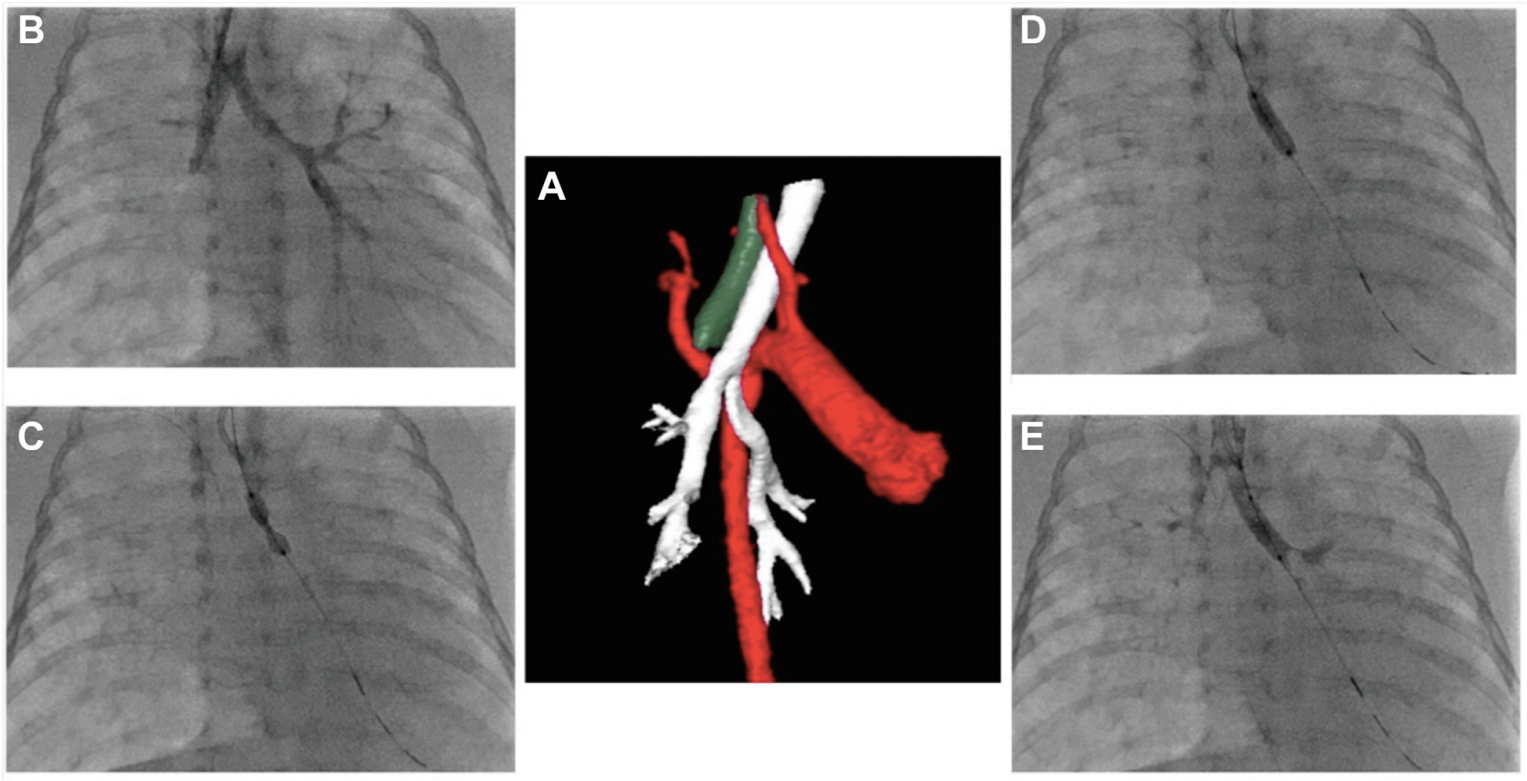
Procedural steps. (A) Three-dimensional segmentation of computed tomography angiogram showing the compressed bronchus in white, aorta in red, and esophagus in dark green. (B) Bronchogram showing the area of narrowing of the distal left bronchus at the take-off of the upper and lower branches. (C, D) Showing balloon inflation of the stent; note the narrowing midstent. (E) Bronchogram after stent placement showing improved caliber of the bronchus.
